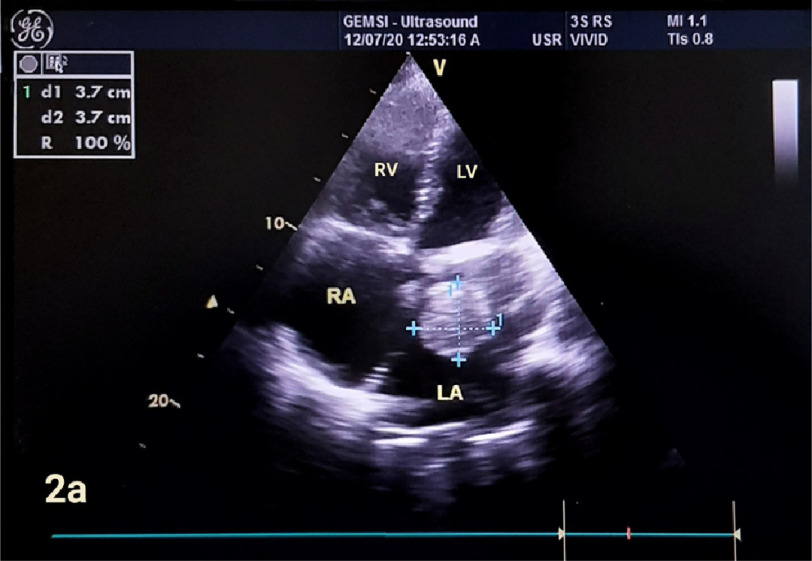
Transthoracic echocardiography. Apical 4-chamber view showed large freely mobile ball thrombus 37 mm in diameter floating in dilated LA. LA =left atrium, LV =left ventricle, RA =right atrium, RV =right ventricle.
